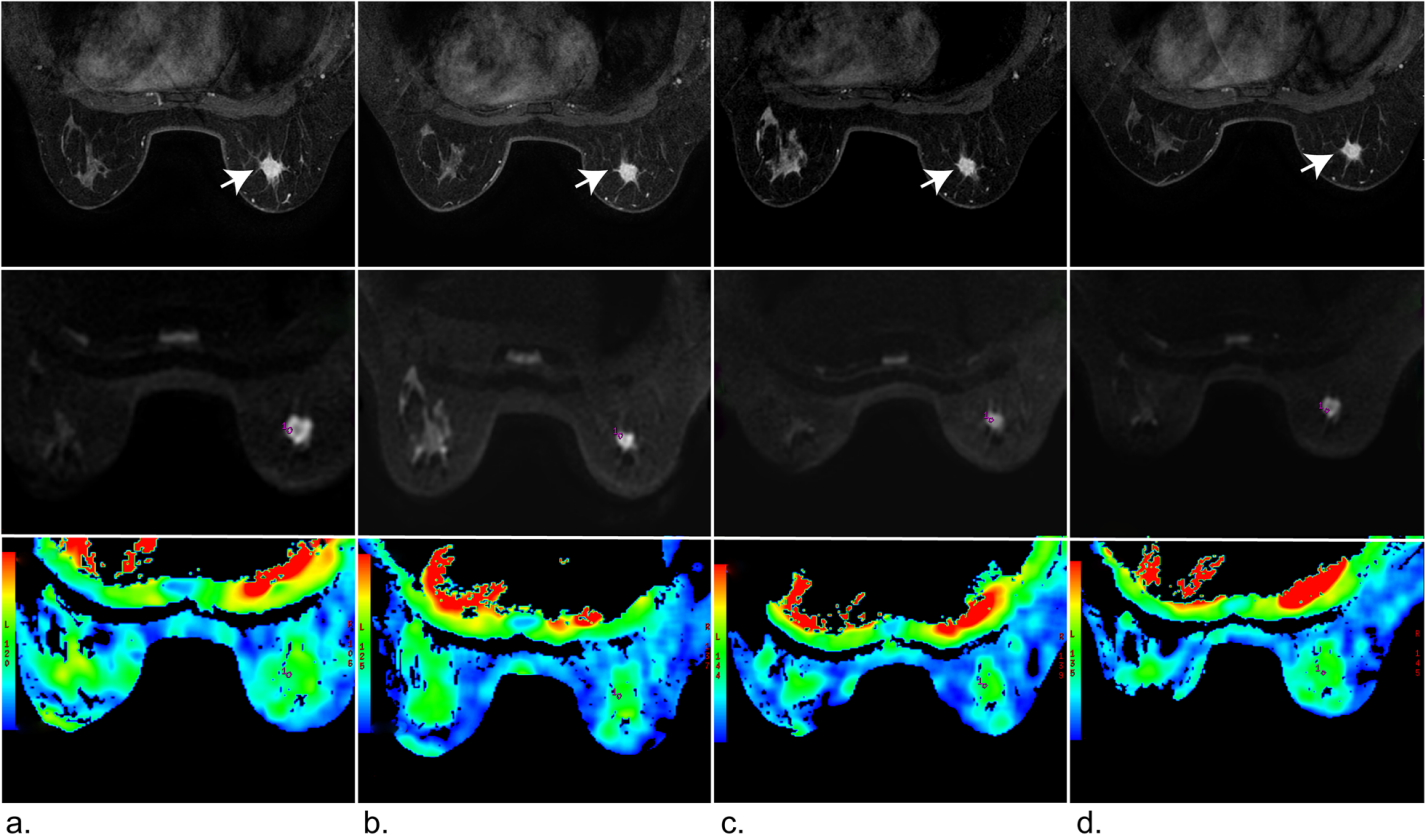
MRI images of a 51-year-old woman with invasive breast cancer who showed non-pCR after completing neoadjuvant chemotherapy (four cycles EC-T). a–d From top to bottom, the images show the vibrant enhancement sequence, IVIM DWI, and ADC map, respectively. The ROI area of the DWI images is marked by a red circle. At baseline, first follow-up, second follow-up, and preoperatively, the tumor volume (white arrow) was 2.40, 2.10, 2.08, and 2.09 cm^2^, respectively, and the standard ADC value was 1.21 × 10^−3^, 1.18 × 10^−3^, 1.20 × 10^−3^, and 1.22 × 10^−3^ mm^2^/s, respectively
